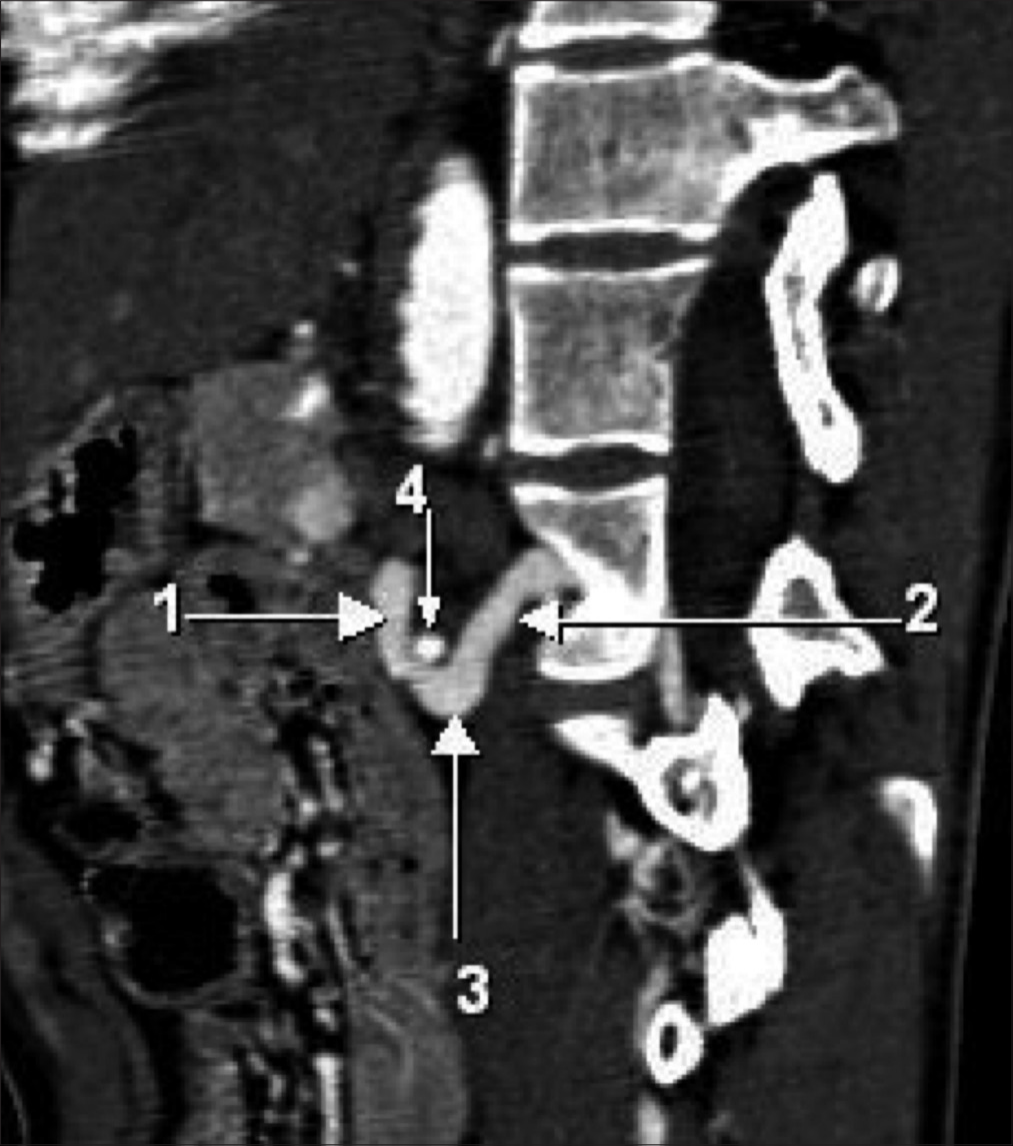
Sagittal reformatted maximum intensity projection CT scan shows the varicosity of the communicating vein between the left renal vein and the left ascending lumbar vein straddling the left renal artery. (The arrows point to the same structures as in Figure 3)
